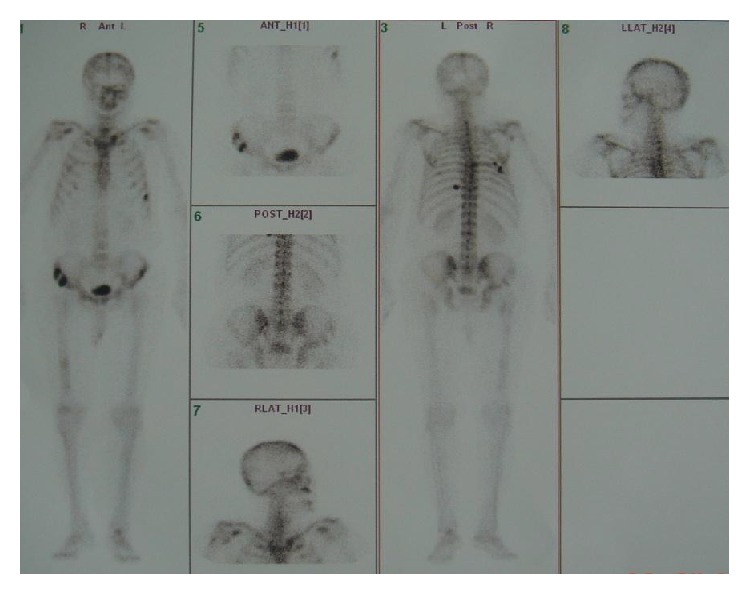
Bone scintigraphy, intense activity in multiple bones.
